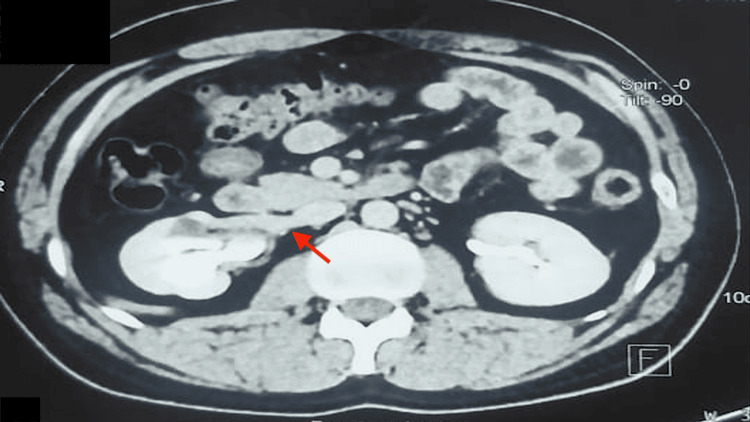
Multidetector CT scan showing compression of the right ureter by the crossing tributary of IMV, causing upstream hydroureteronephrosis IMV: inferior mesenteric vein
